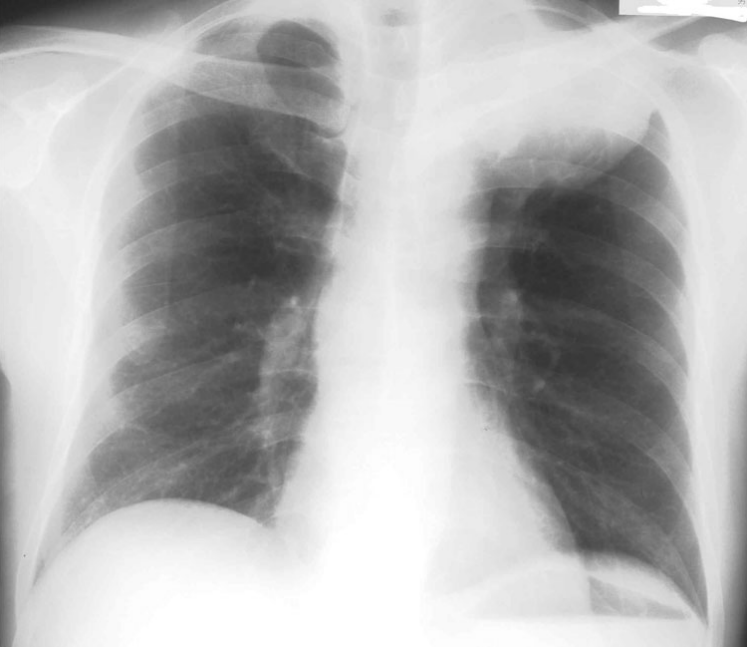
Posterior-anterior view of a chest X-ray film demonstrated a huge mass shadow in the left upper lung field.
